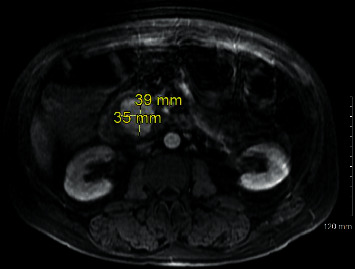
Magnetic resonance imaging with measurement of pseudoaneurysm.
